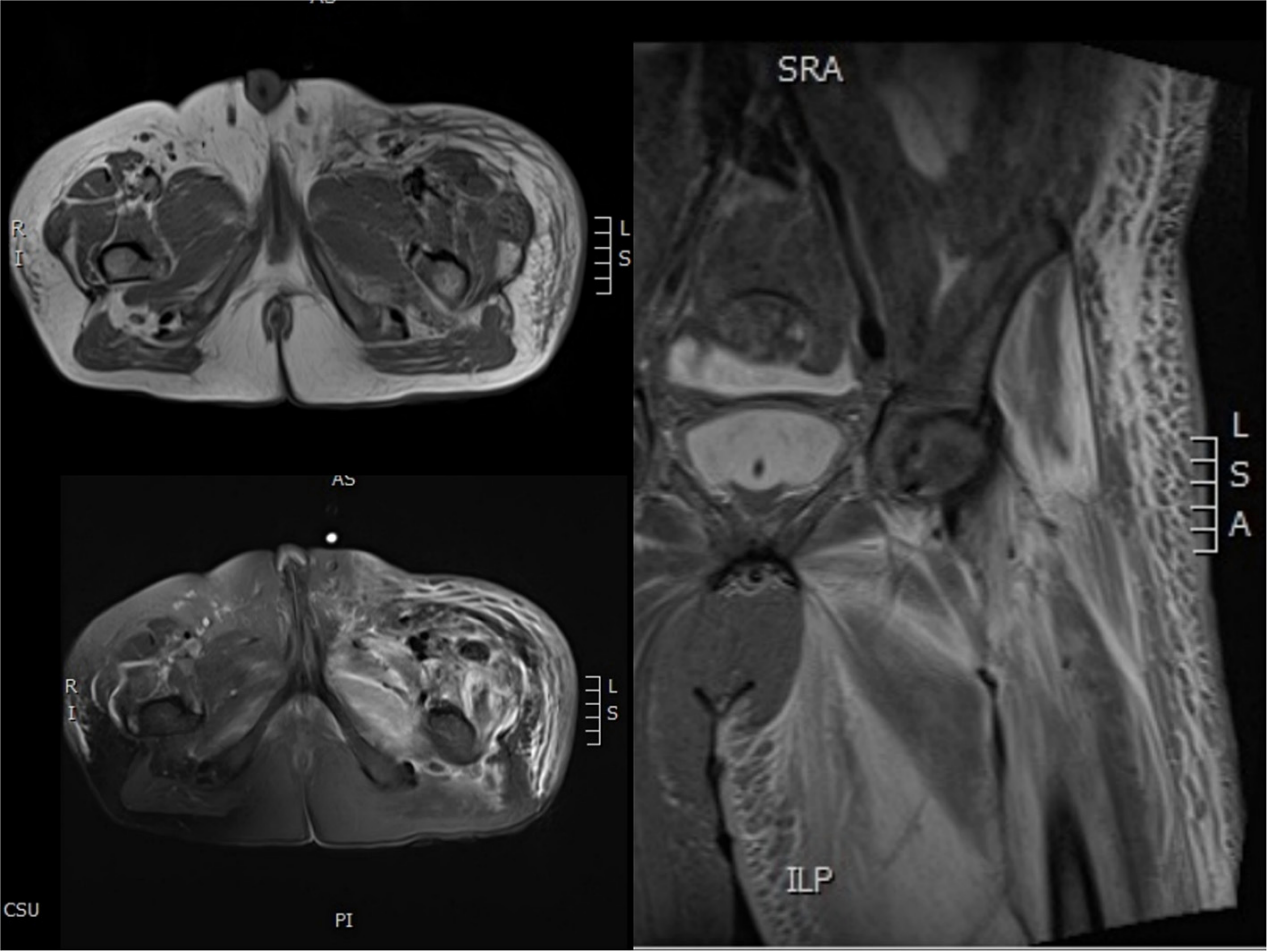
MRI plain scans of the left lower limb.
